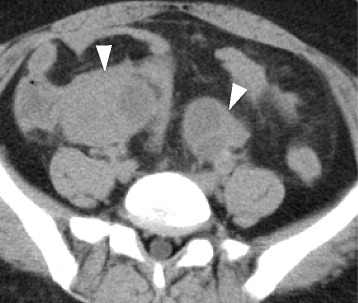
Abdominal computed tomography revealed thick-walled multilocular cystic lesions at the bilateral ovaries (*white arrowheads*)
